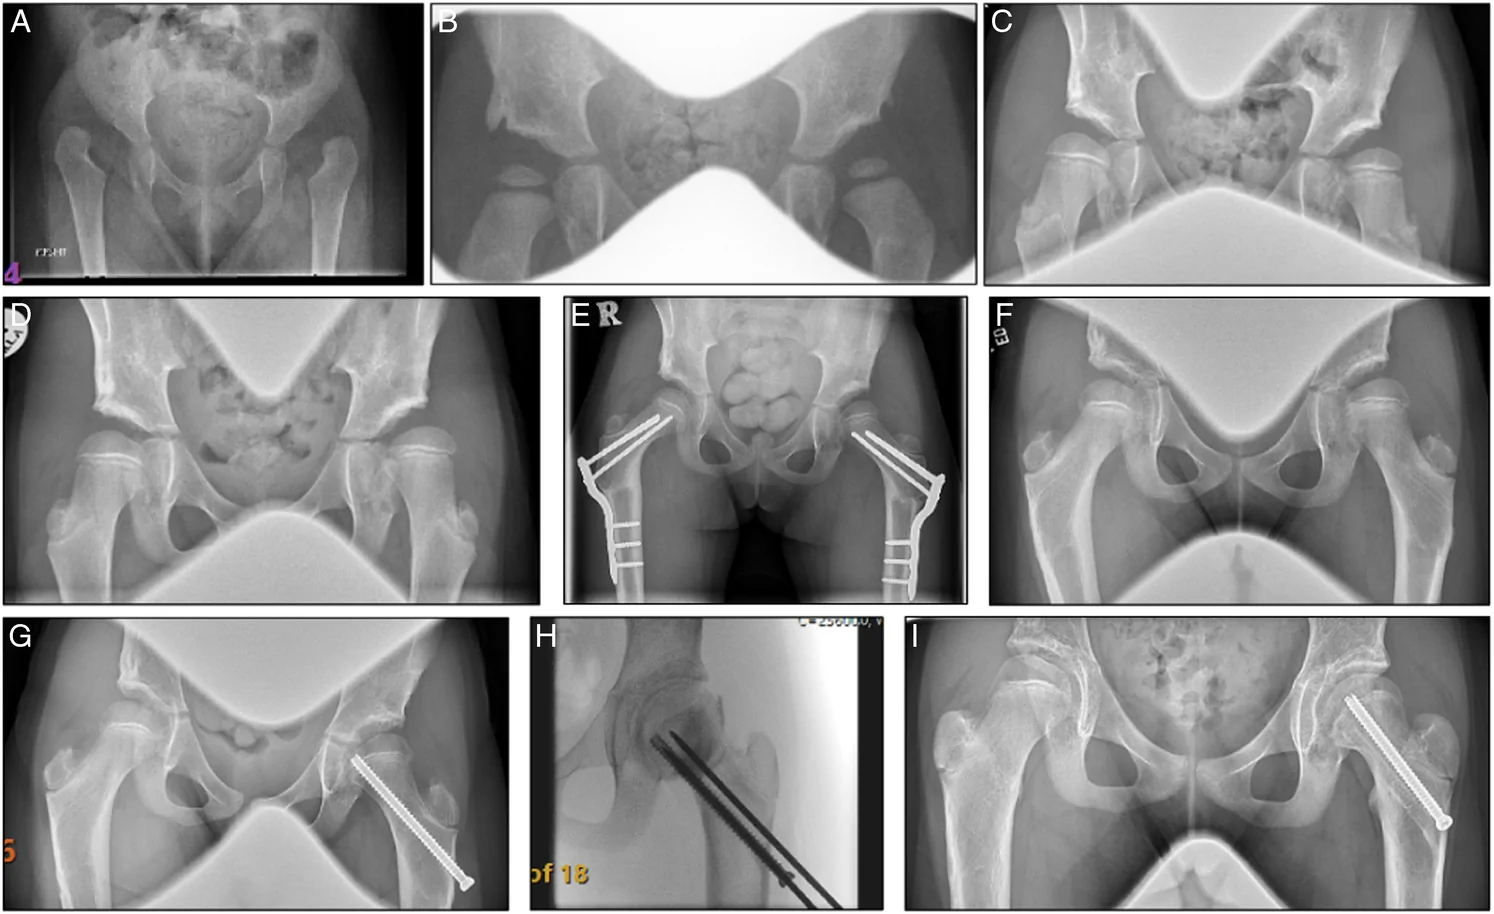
Serial radiographs following bilateral sequential hip open reduction and growth-stimulating minimal acetabuloplasty, at 11 and 16 months of age, plus subsequent further corrective surgery (FCS) for lateral growth arrest. (A) Preoperative showing IHDI 4 hips with AIs of 41 degrees (right) and 40 degrees (left). (B, C, D) At 1, 4, and 6 years following surgery, showing progressive lateral growth arrest and an increase in neck-shaft angle (NSA), left worse than right. Despite this hip displacement, the acetabulae have continued to mature. (E) Three months following bilateral FVO, demonstrating restoration of concentric position of both hips. (F) Recurrence of increased NSA on the left side at 2 years following FVOs. (G) A medial femoral head screw was placed to halt further progression at 9 years following index surgery. (H) An exchange screw was placed after the first screw had grown off the physis at 11 years postoperatively. (I) Twelve years postoperatively: right AI 9 degrees/CEA 36 degrees; left AI 11 degrees/CEA 33 degrees; both hips Severin 2. At 13 years old, the patient is asymptomatic and participating in all sports without limitation.
